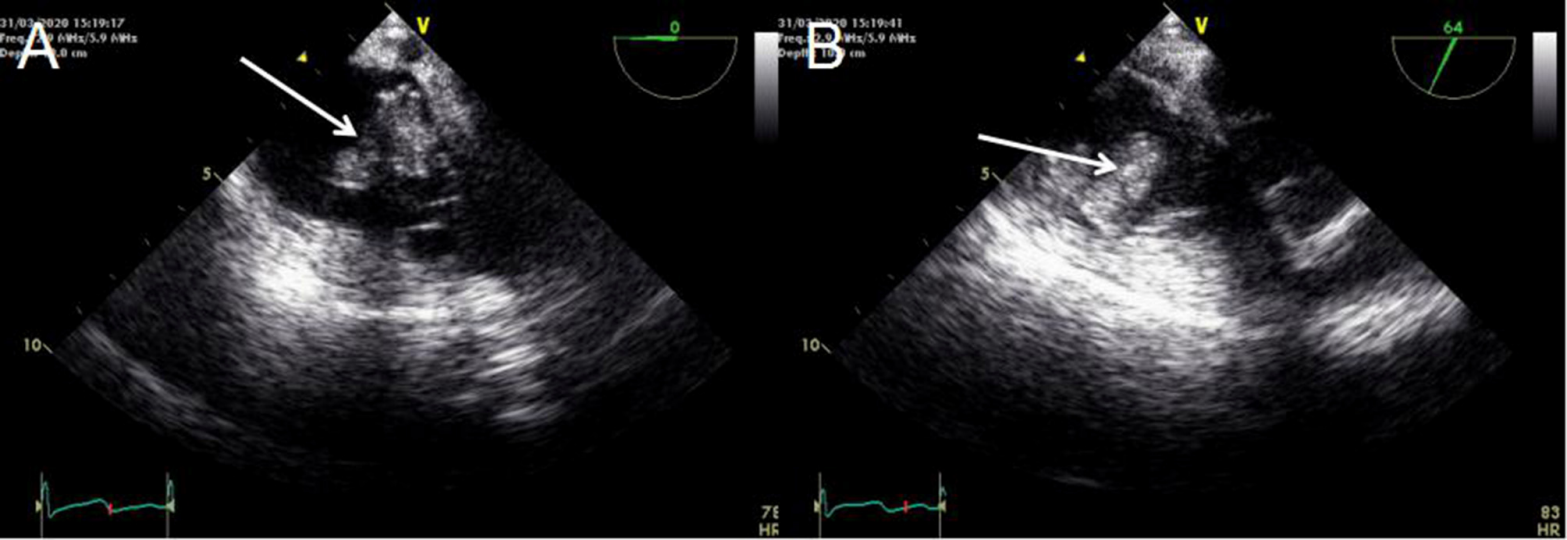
(A,B) Transesophageal echocardiography showing lumpy vegetations in the tricuspid valve.
